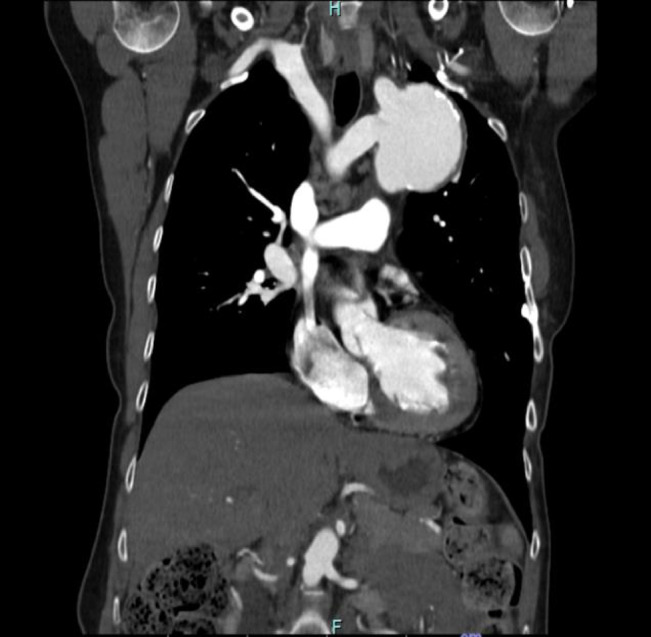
Computed tomography angiography (CTA) showing a large 7.3 cm saccular aneurysm of the distal thoracic aortic arch and proximal descending aorta in the coronal plane
